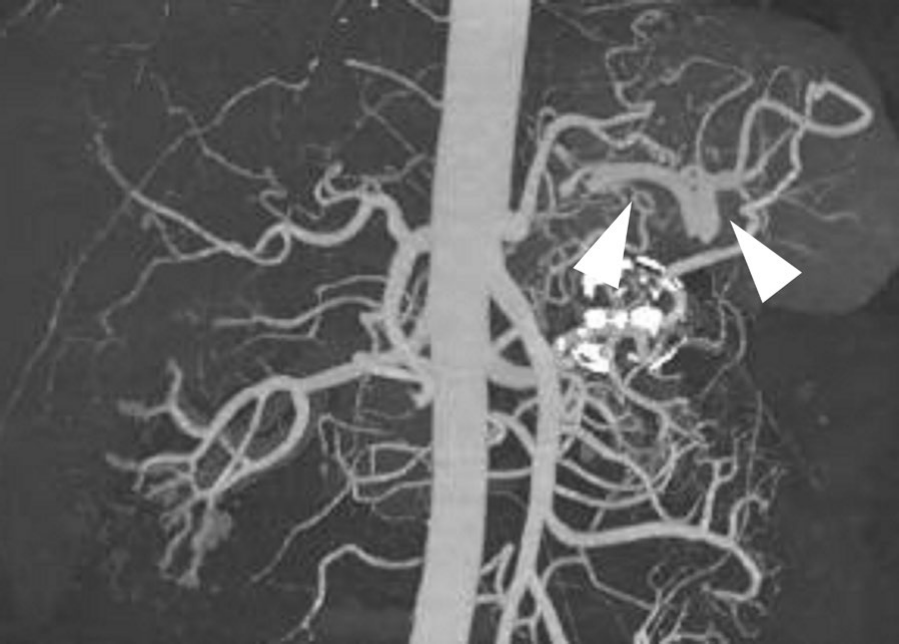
Contrast-enhanced computed tomography scan at 1 month postoperatively. The splenic artery aneurysm completely disappeared, and the distal splenic artery (white triangle) was visible
